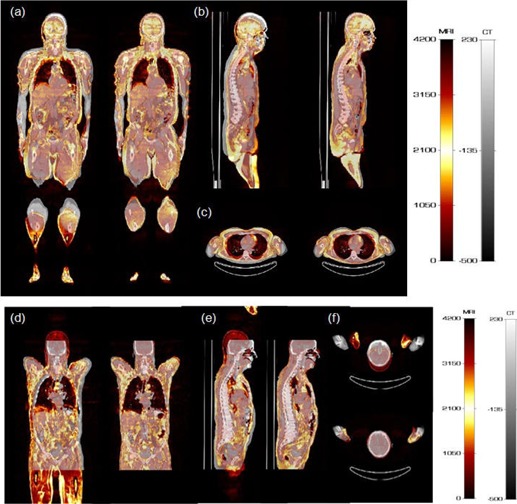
Representative clinical studies of two patients showing 3D fused MRI and CT images before and after image registration: (a) and (d) coronal, (b) and (e) sagittal, and (c) and (f) transaxial views. For each image pair, left and right panels represent the same image before and after registration, respectively.
